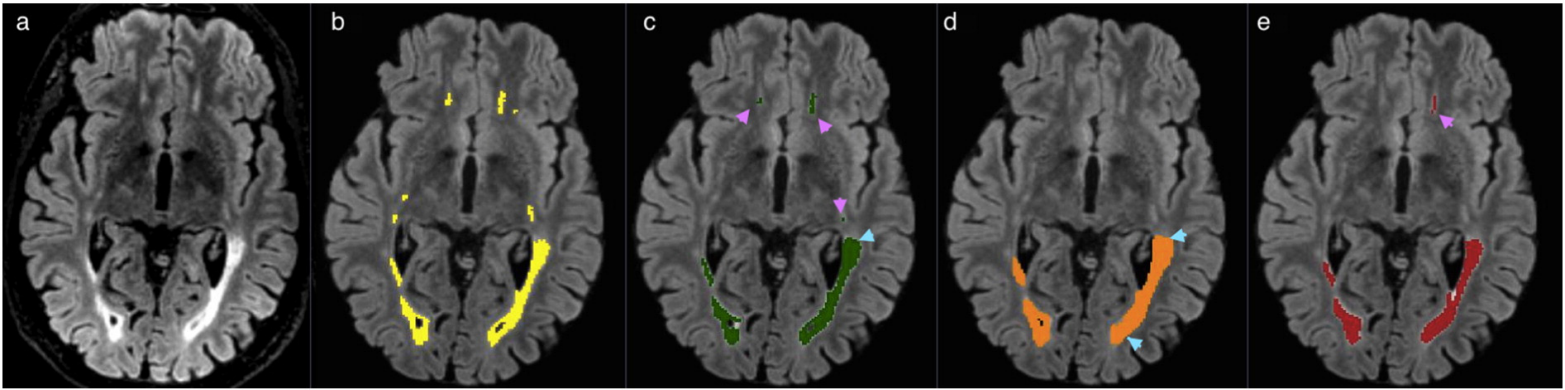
Original FLAIR image (a) followed by bias corrected FLAIR image and super-imposed lesion segmentation from: (b) expert segmentation, (c) MSmetrix, (d) LST, and (e) Lesion-TOADS. Cyan arrow heads show false positive lesions and overestimation of the lesion boundaries in LST. Pink arrow heads show lesions picked by MSmetrix but not by the other methods except one in Lesion-TOADS.
